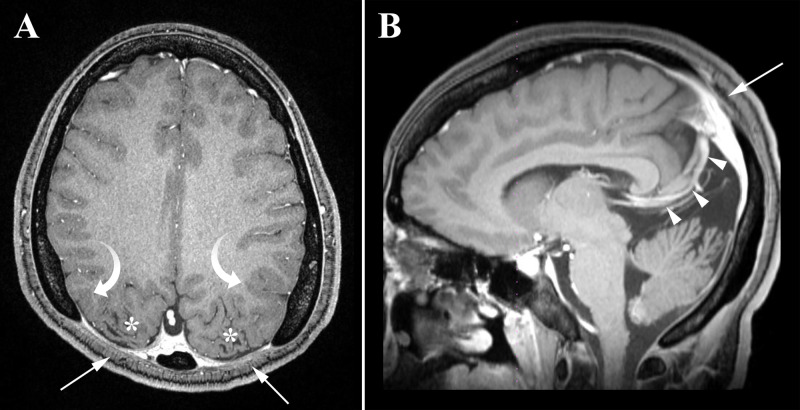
Axial (A) and sagittal (B) post-contrast T1-weighted MRI images show large parietal bone defects (arrows) with underlying cortical malformations including ulegyria (asterisks) and polymicrogyria (curved arrows). A persistent falcine sinus (arrowheads) drains the deep venous system to the superior sagittal sinus and the straight sinus is absent. The falcine sinus is a normal fetal vein that ordinarily involutes after birth. Additionally, there is a large posterior fossa with a high insertion of the tentorium cerebri.
